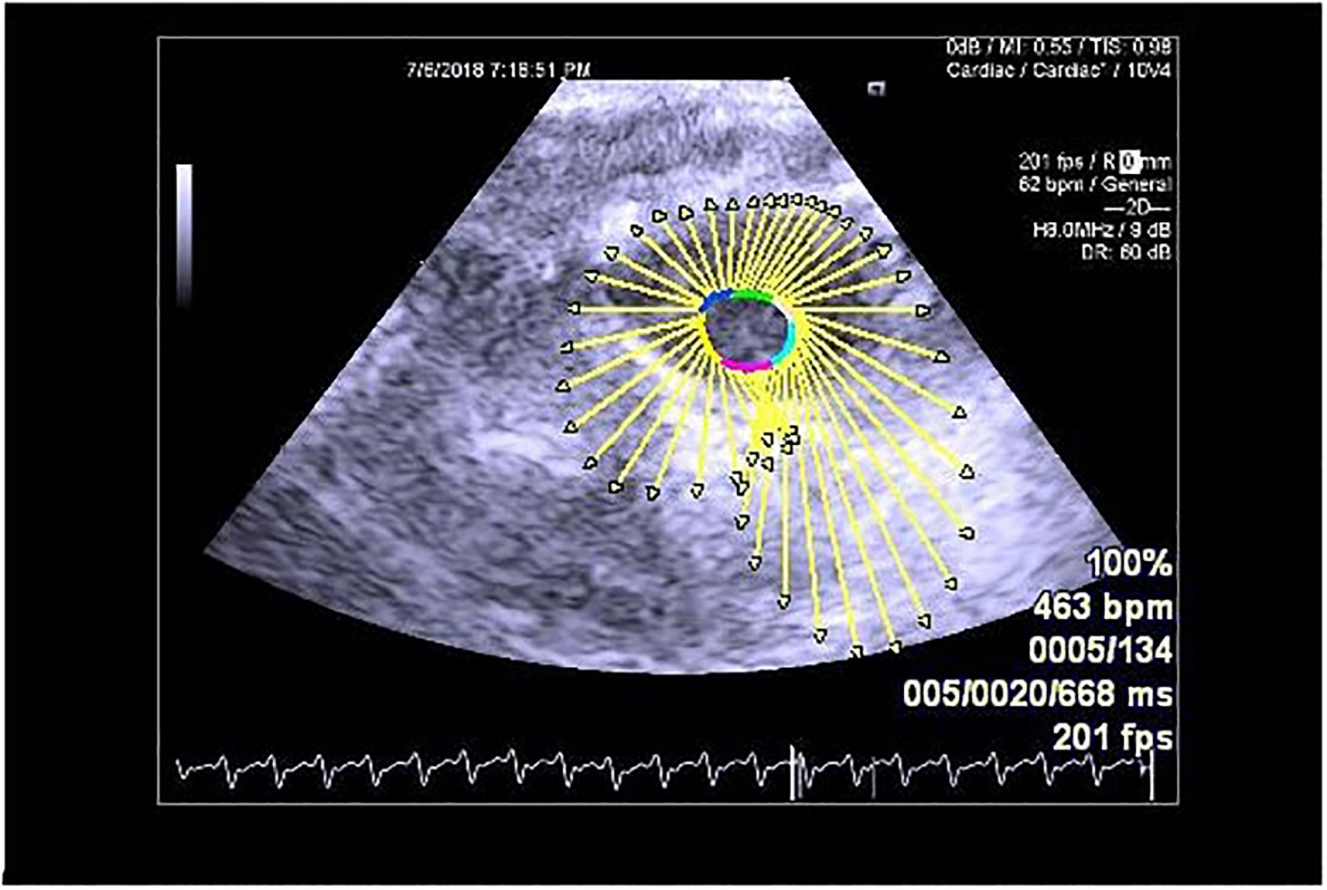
VVI technology to analyze parasternal short axis mitral valve.
